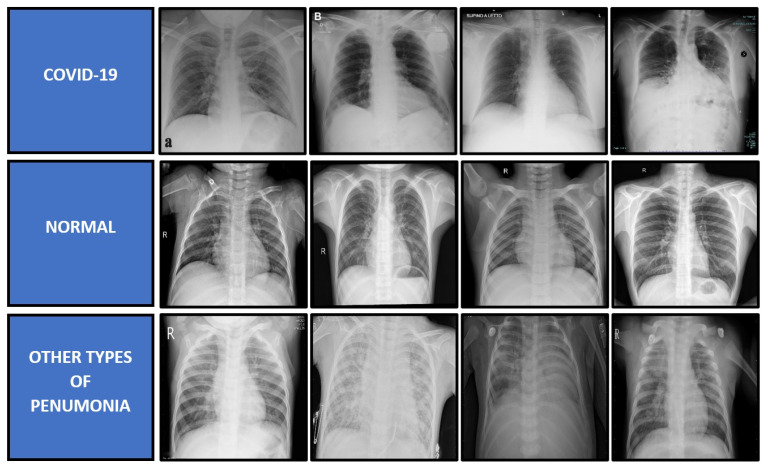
Samples of X-ray images for each category of COVID-19, normal and other types of pneumonia cases.
